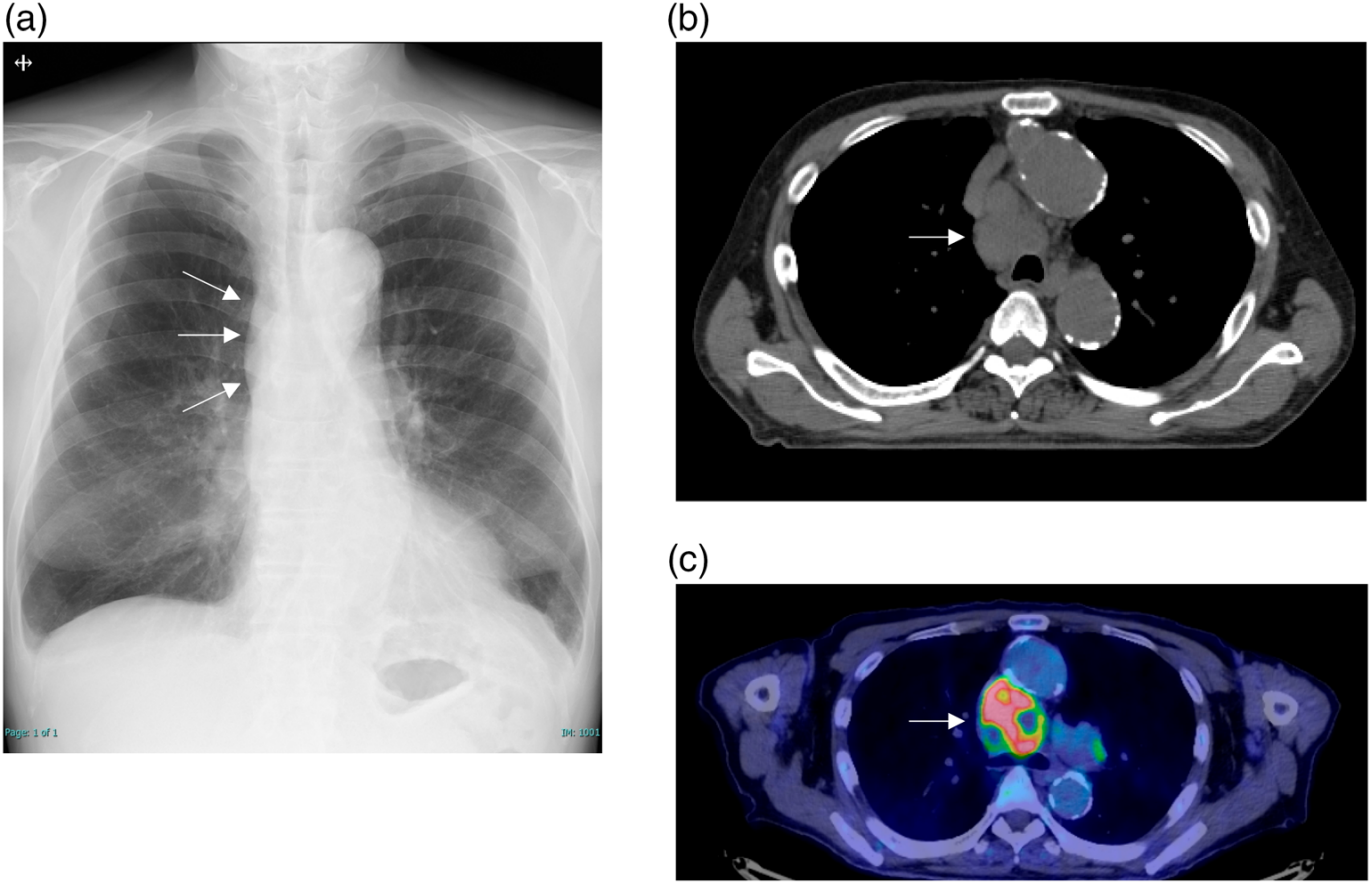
Radiological imaging at initial diagnosis. (a) A chest x‐ray showed an enlarged hilar shadow on the right side. (b) A computed tomography showed an enlarged right anterior paratracheal lymph node. (c) 18F‐fluorodeoxyglucose‐positron emission tomography showed a highly enhanced signal in an enlarged paratracheal lymph node. White arrow: metastatic paratracheal lymph node.
